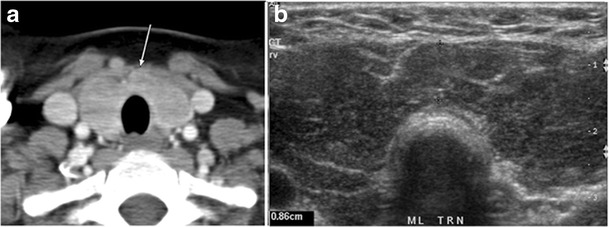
A 33-year-old female patient who presented with neck swelling and pain and was later diagnosed with Hashimoto’s thyroiditis. a Axial enhanced CT scan of the neck demonstrates minimal diffuse enlargement of the thyroid gland, especially the isthmus (*white arrow*). b Transverse greyscale ultrasound of the neck demonstrates heterogeneously enlarged thyroid and thickened isthmus, measuring 8.6 mm
